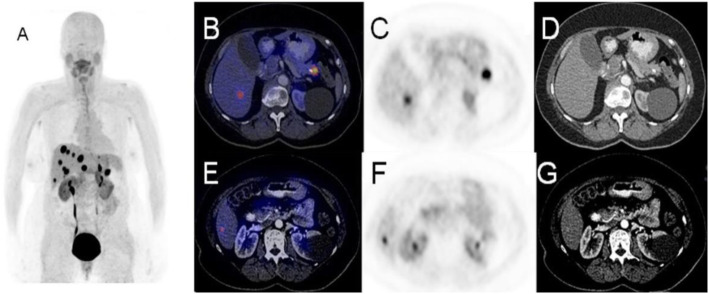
^11^C 5-HTP NET of the pancreas, staging. *Clinical history*: 65 y.o. woman with pancreatic NET, metastatic to liver. *PET/CT findings*: MIP (a) showing multiple sites of intense tracer accumulation in the upper abdomen. Fused images of PET with diagnostic CT (b, e), PET only (c, f), and CT only (d, g) showing intense tracer accumulation in a lesion in the cauda of the pancreas, with vague contrast enhancement and a calcification on the CT as well as in liver metastases (b–d)
